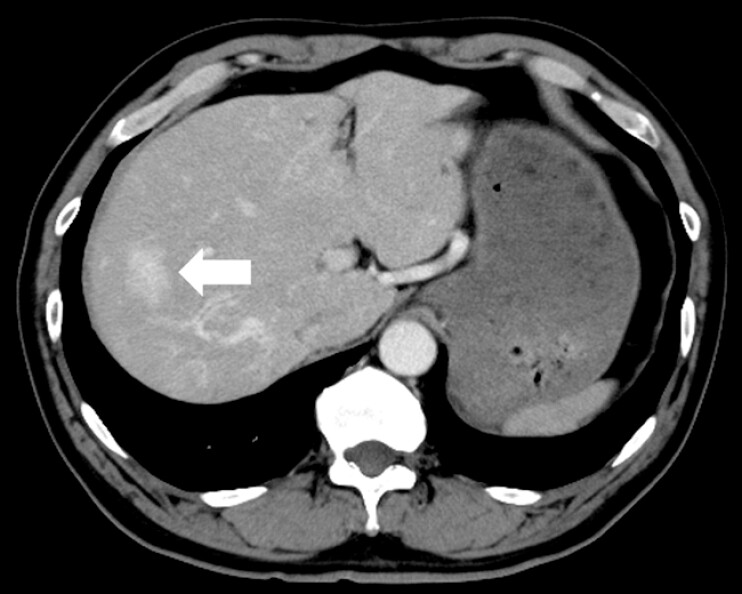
Contrast-enhanced computed tomography showing a hepatic mass with peripheral contrast enhancement, suspected to be a hemangioma.
